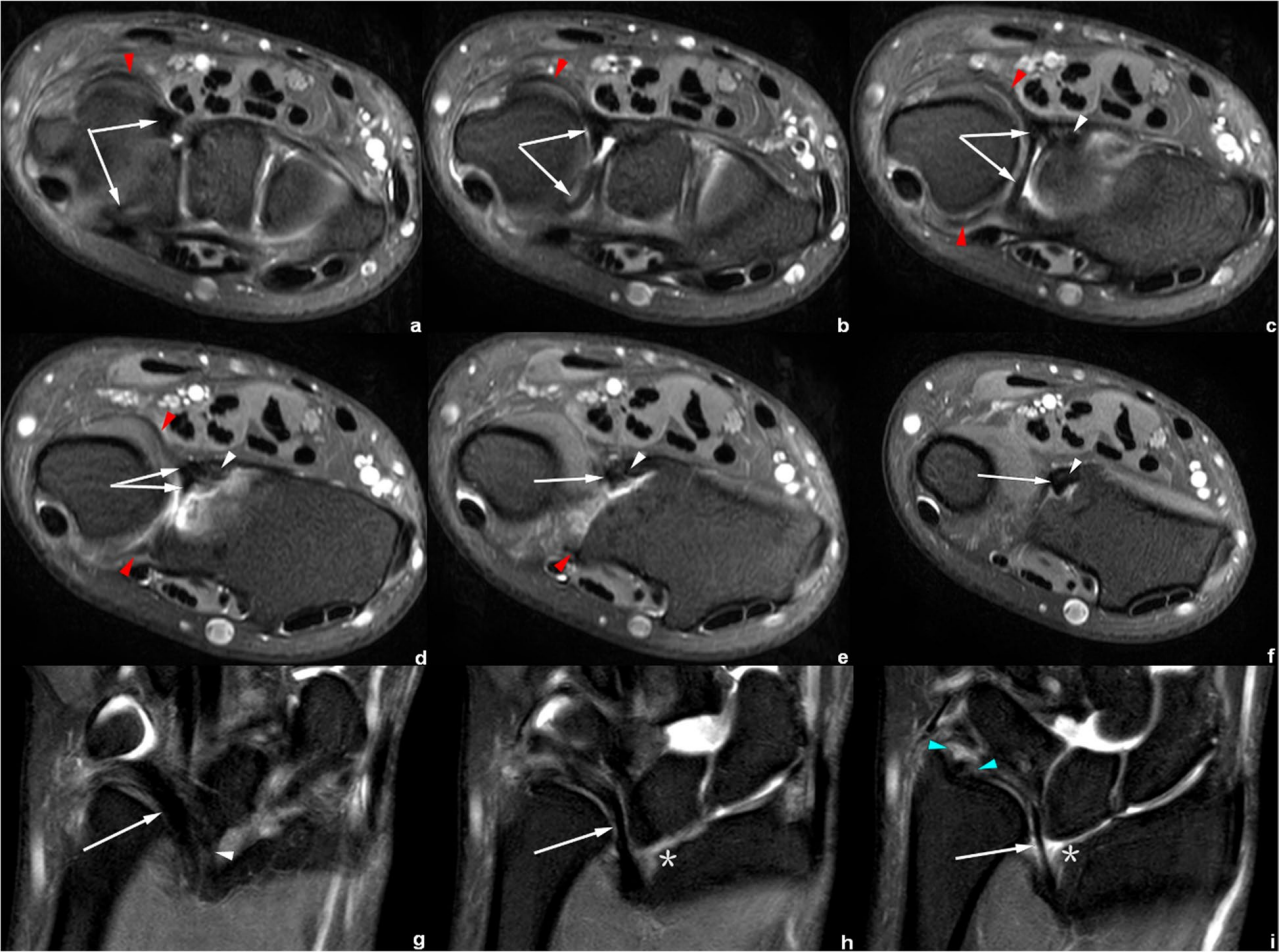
Right wrist MRI of the patient No. 1018. a–f Axial PDWI-FS images show the CD (white arrow), volar and distal radioulnar ligament (red arrowhead) and VL (white arrowhead). g–i Coronal PDWI-FS images show the VL (white arrowhead) and a tilted, thickened CD (white arrow) with the radial insertion significantly shifted proximally from the medial rim of distal radial articular surface (*). The blue arrowheads demonstrate the distal and proximal triangular ligaments, which attached the CD to the distal ulna
